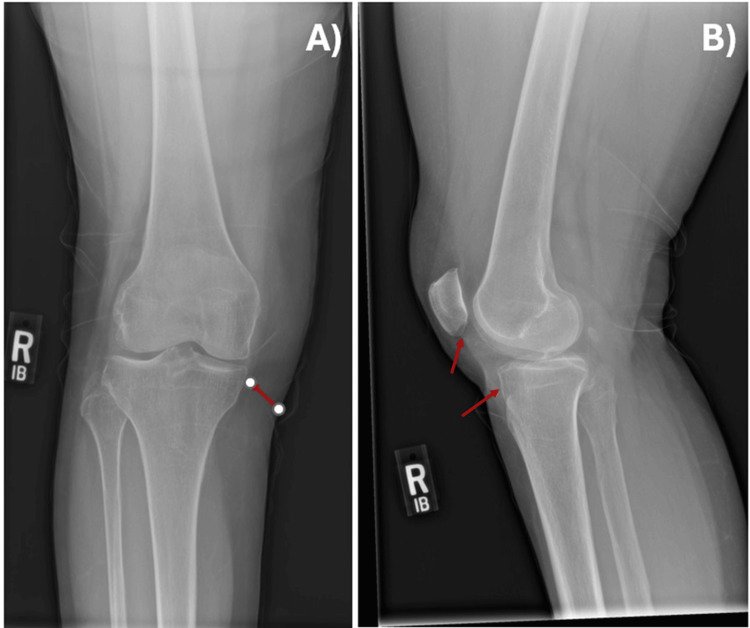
X-ray of the right knee in frontal (A) and lateral (B) views, with red arrows highlighting areas of cortical thinning.
